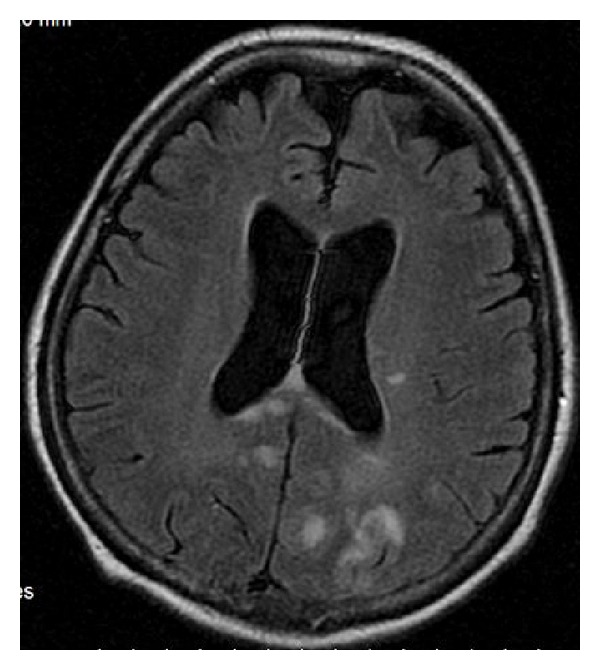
FLAIR, axial plane. Posterior reversible encephalopathy syndrome (PRES) in a 60-year-old woman with renal carcinoma and severe hypertension.
